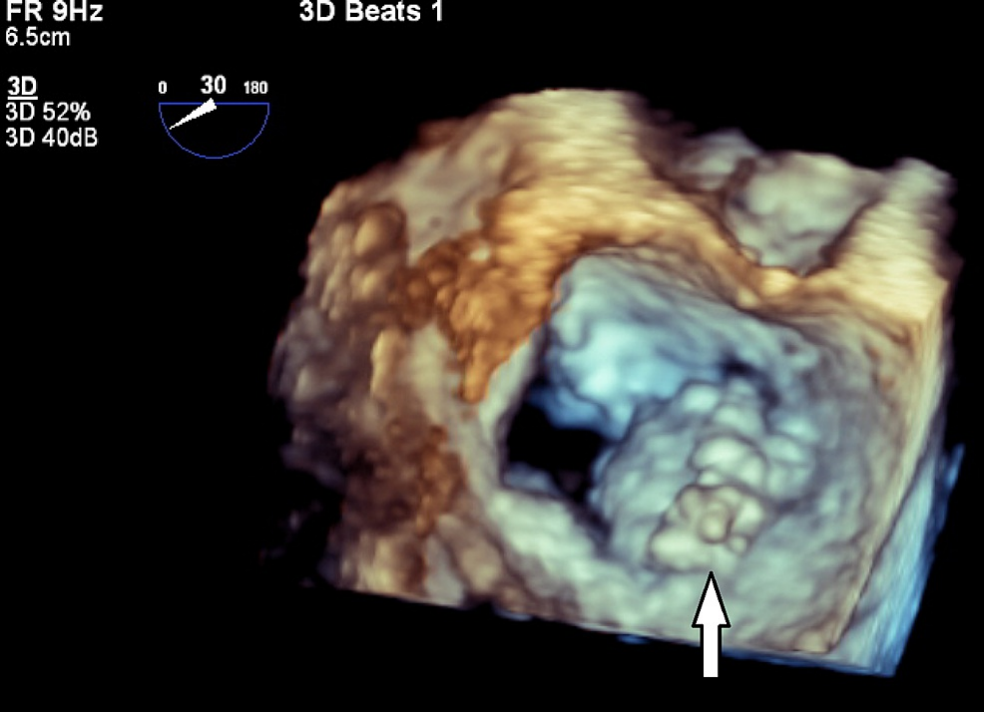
Transesophageal echocardiogram with a three-dimensional (surgeon’s view) image displaying a vegetation (white arrow) on the mitral annulus
